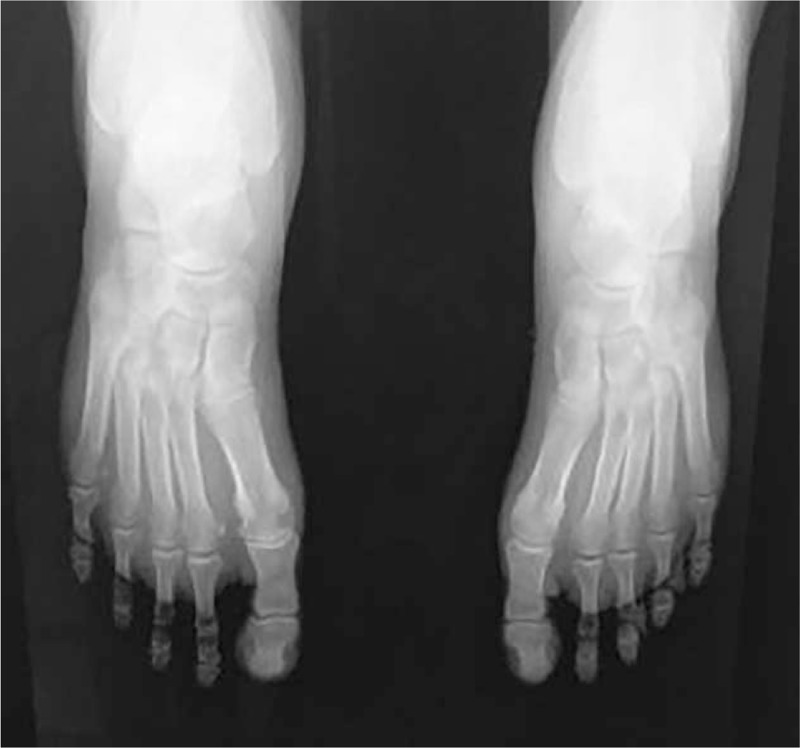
Radiographic examination of the lower limbs.
